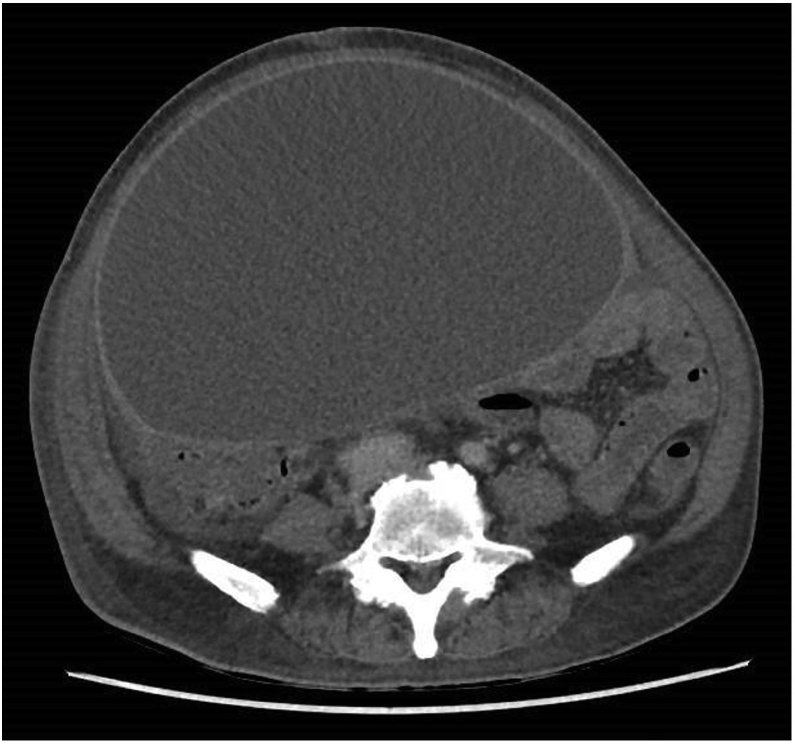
Contrast CT (axial view) showing large well-circumscribed homogenous hypoechoic cystic lesion with mass effect.
